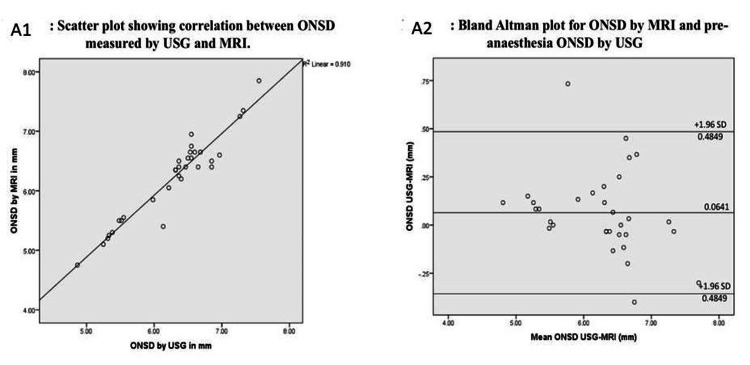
Correlation between ONSD measured by MRI and USG. (A1) Scatter plot; (A2) Bland-Altman plot ONSD: optic nerve sheath diameter; MRI: magnetic resonance imaging; USG: ultrasonography
